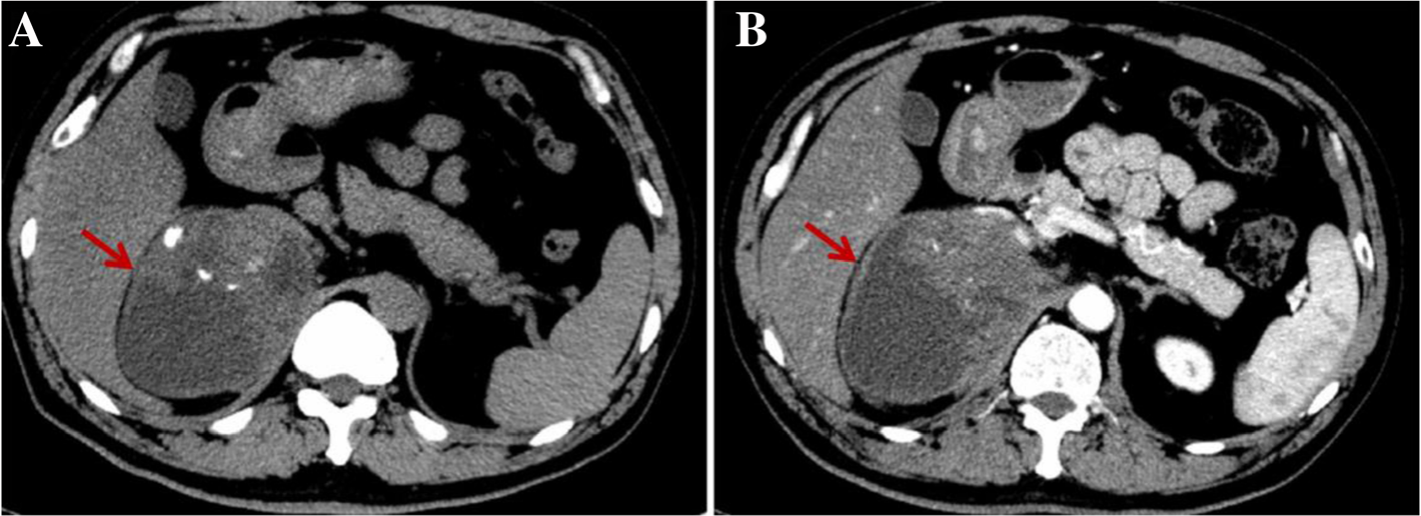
Abdominal computed tomography (CT) scan revealed a large mass (*arrow*) arising from the right adrenal gland (a). The lesion showed heterogeneous enhancement and relatively sharp margination (*arrow*) on Contrast-enhanced CT (b)
